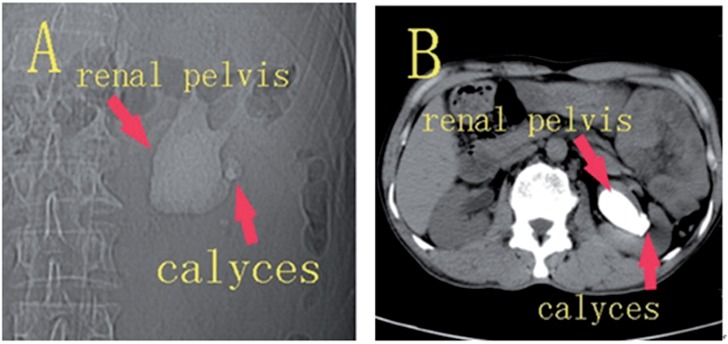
Non-contrast computed tomography.
